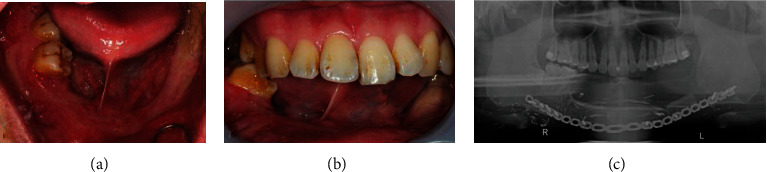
The soft and hard tissues had healed completely and ready for reconstruction. (a, b) Intraoral view. Teeth 46 and 47 were temporarily kept for maintaining vertical support. (c) Panoramic radiograph after segmental mandibulectomy and fibular free flap.
